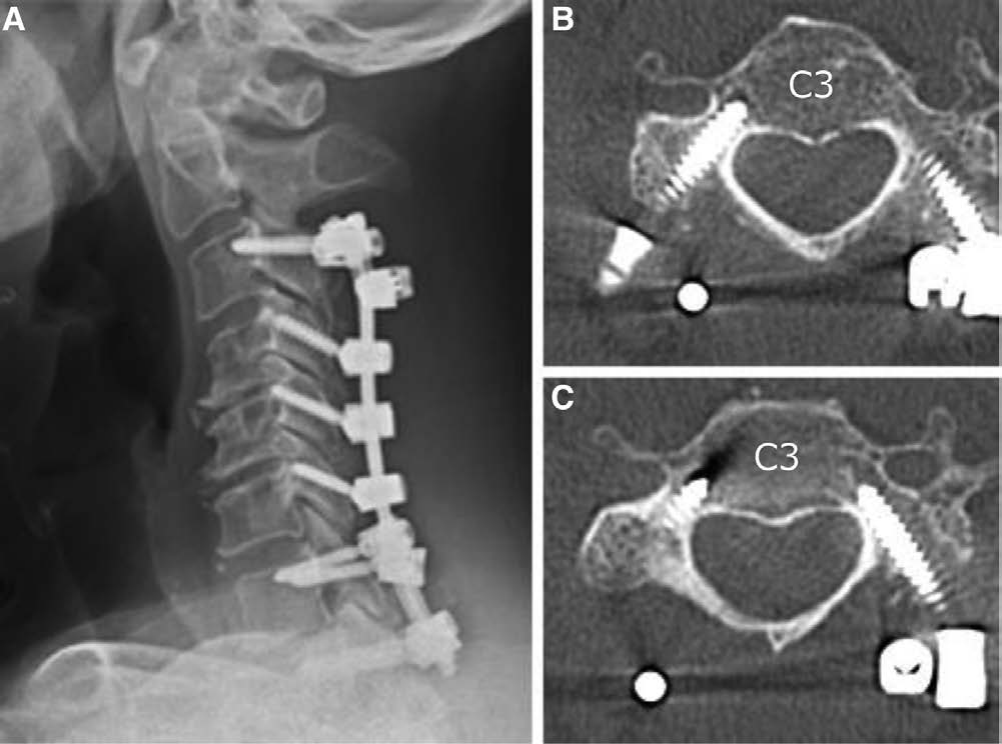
Images 1 month following surgery. (A) Neutral plain X-ray of the lateral side of the cervical spine. (B and C) Postoperative CT transversal images.
